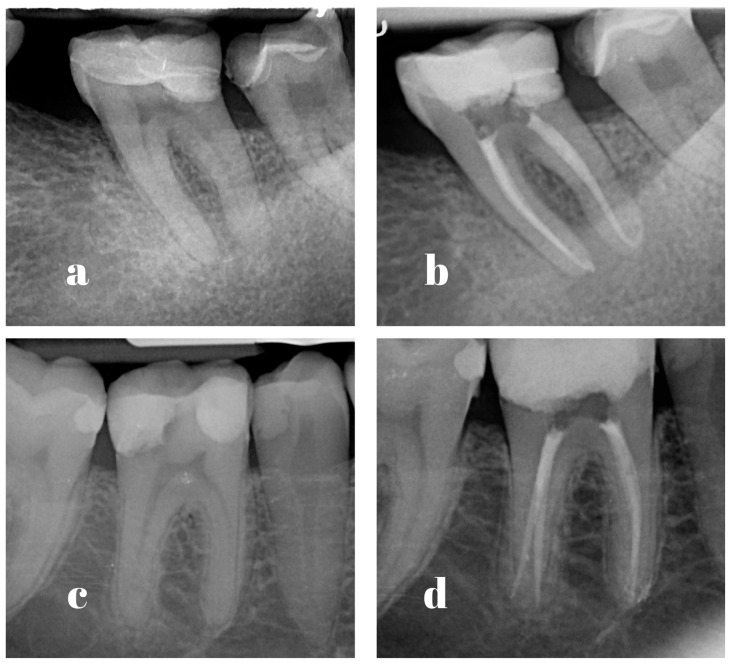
Examples of cases performed by PGSs ((a) pre-operative radiograph; (b) post-operative radiograph) and ESs ((c) pre-operative radiograph; (d) post-operative radiograph).
